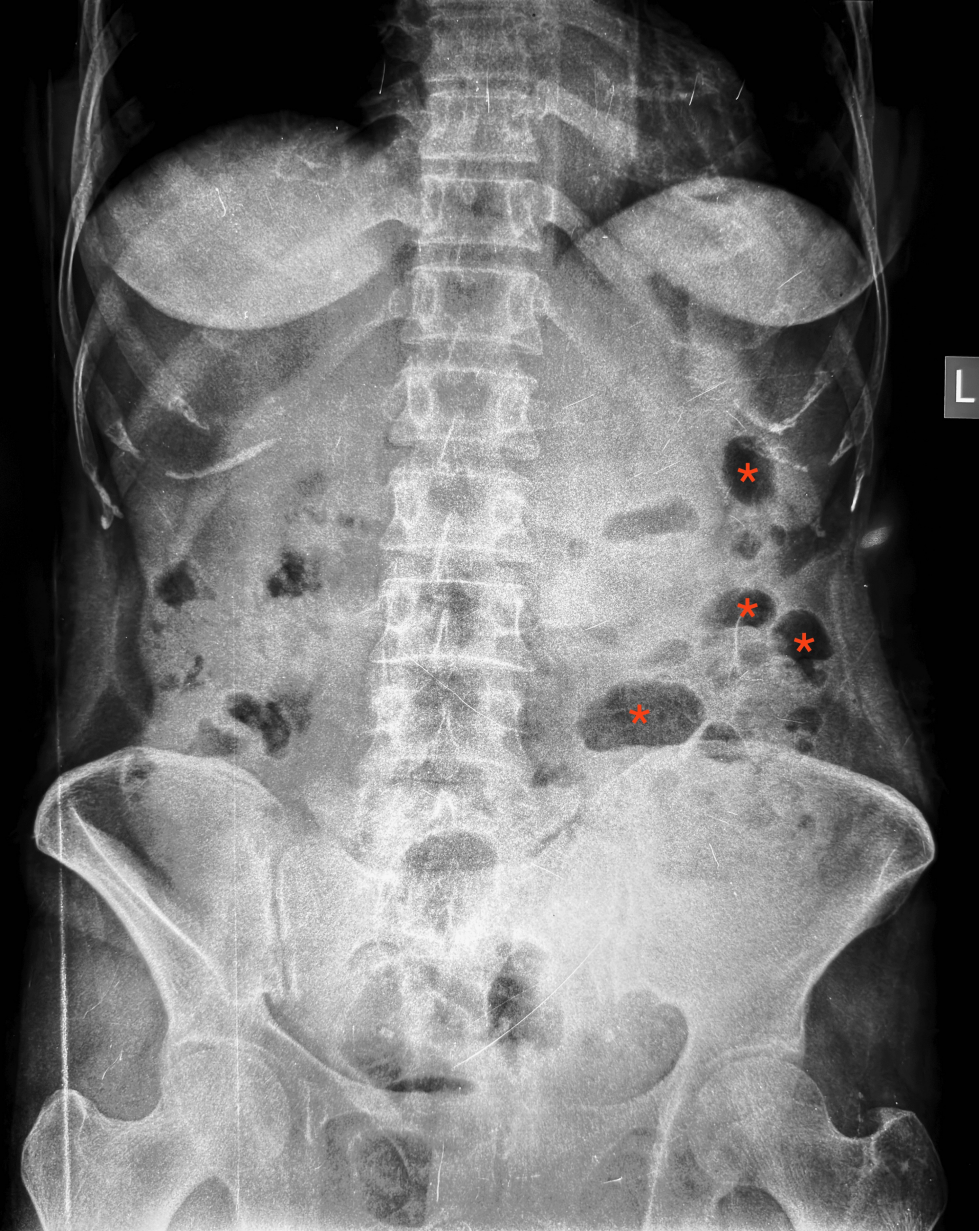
Plain abdominal radiograph showing dilated bowel loops (marked with *) consistent with obstruction
